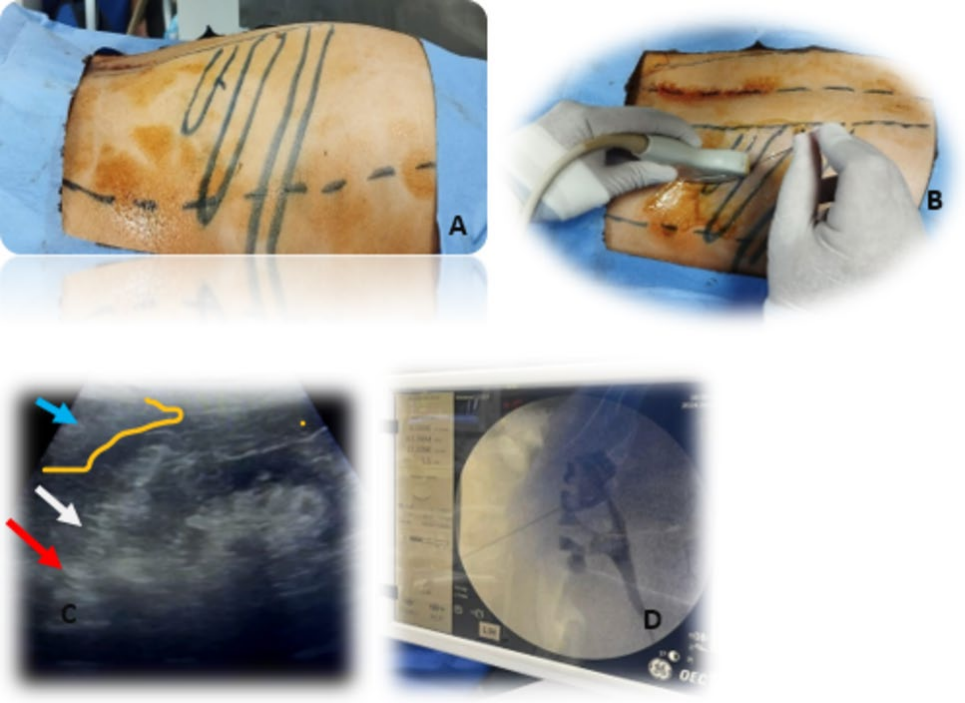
Demonstrates SUGA-PNL technique. A; shows the surface landmarks of last 3 ribs and the posterior axillary line, B; puncture needle placement supra-costal approach guided with ultrasound, C; ultrasound image shows the needle puncture through the targeted upper calyx harboring the stone, red arrow is the stone, white arrow is the puncture needle, blue arrow and yellow outline showed lung base, D: Fluoroscopic image of retrograde pyelography after needle placement in the targeted upper calyx. Notably, these figures are archived photos which were taken during the study, compiled by *Ahmed Assem*, ahmed.assem@cu.edu.eg, with all rights preserved
